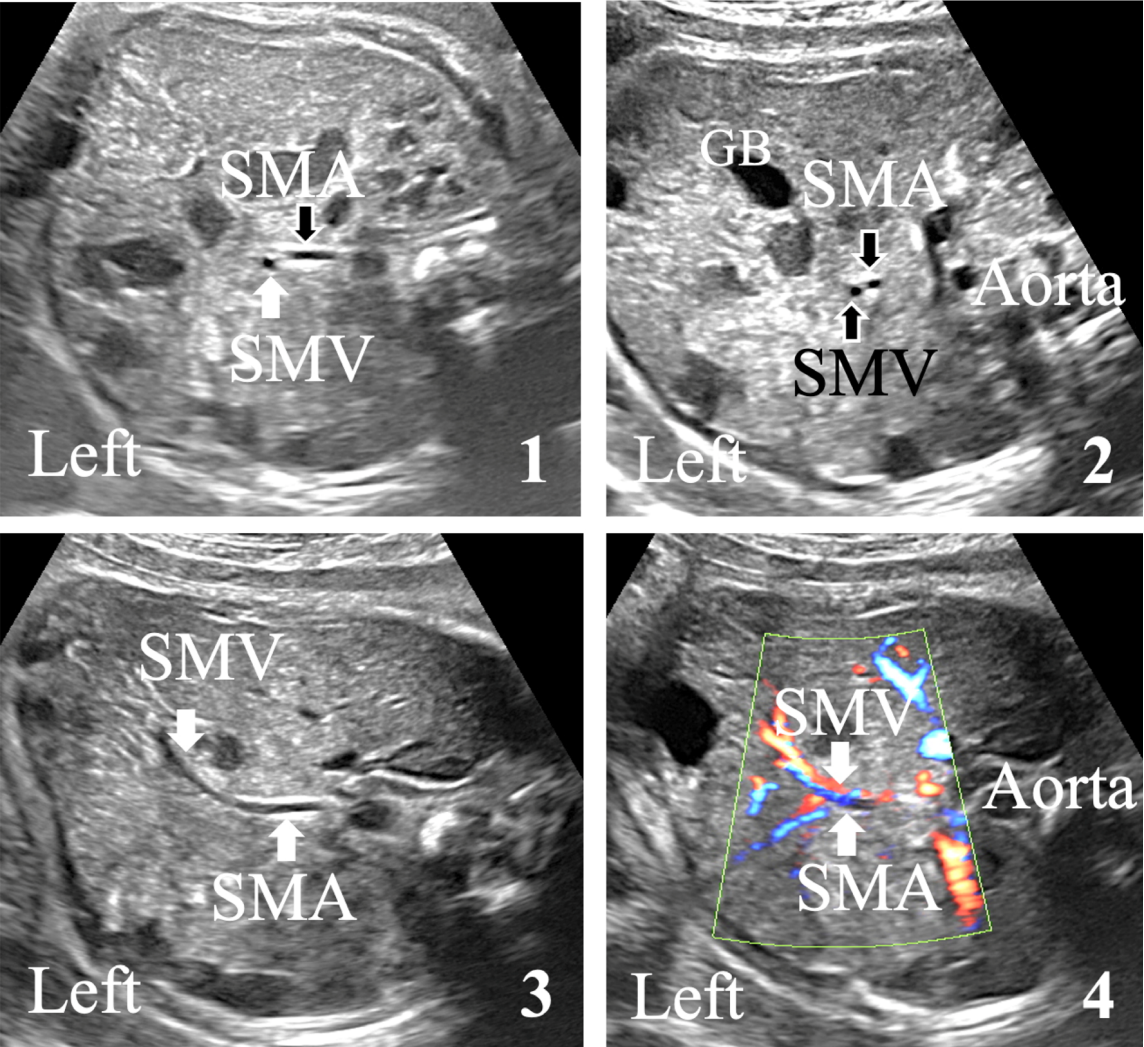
Prenatal ultrasound imaging of the fetal abdomen (transversal view). Prenatal ultrasound of false positive case. The SMV appears initially on the left (1), then in front of the artery (2). The branches of mesenteric vessels have a normal pathway to the right part of the fetal abdomen (3). Color Doppler mode (4) offers correction of technical errors. GB, gallbladder.
